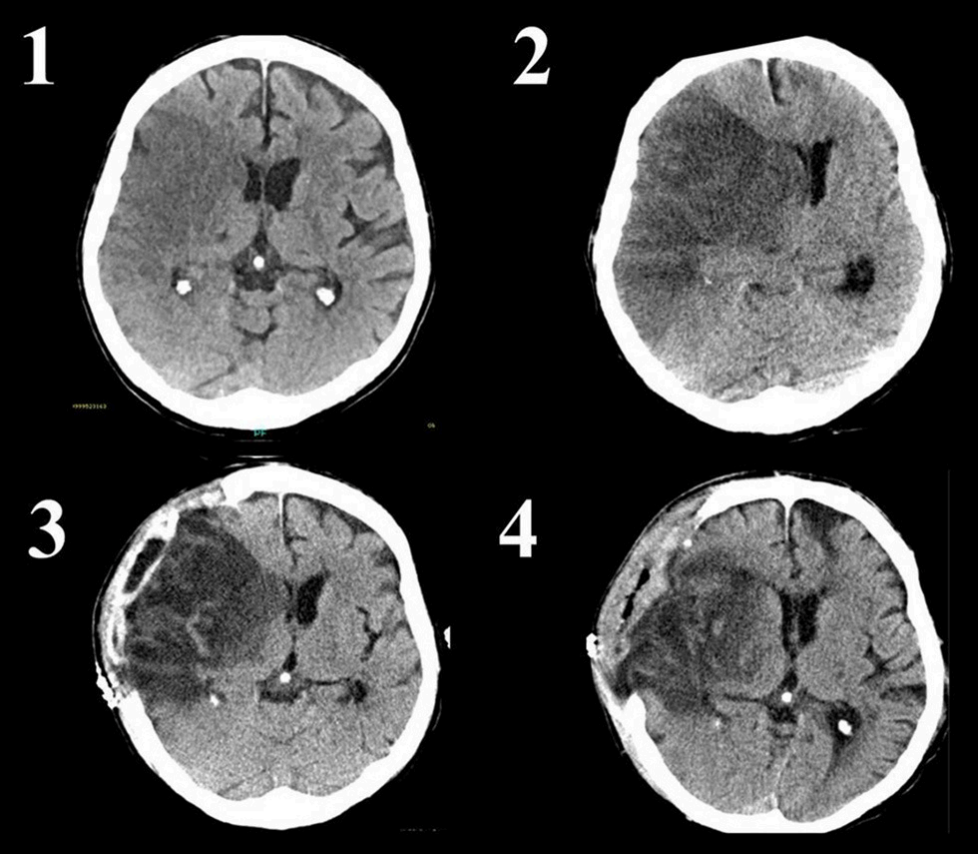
Native CT scans of a patient with infarction of the complete right middle cerebral artery territory. A systemic or endovascular therapy was not conducted due to late arrival and already visible ischemic changes. Image 1 shows the ischemic tissue as darker (hypodense) area without significant mass effect. Image 2 reveals progressive edema of the ischemic tissue with visible midline shift. The occipital horn of the left lateral ventricle is enlarged due to disturbance of cerebrospinal fluid circulation. The patient was alert but deteriorated with reduced level of consciousness immediately prior the CT. Image 3 shows a CT-scan 1 day after decompressive craniectomy. The midline shift and enlargement of the left occipital horn are decreasing, whereas brain tissue is herniating through the skull defect. Image 4 is a CT scan 8 days after decompressive surgery. Midline shift has nearly normalized. The now visible defect on the left frontal part of the brain is caused by an old injury of the patient.
